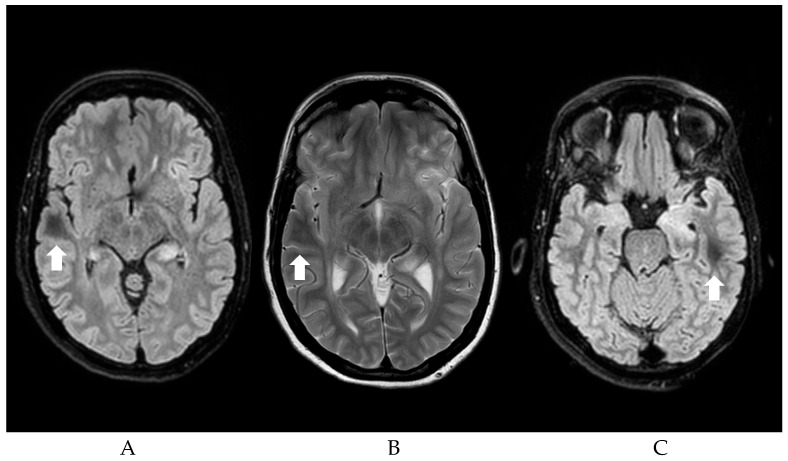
(A,C) FLAIR sequences showing Subcortical localization of DWM (Dark White Matter, see arrows) of a patient with NCSE; (B) T2 Sequences showing DWM.
